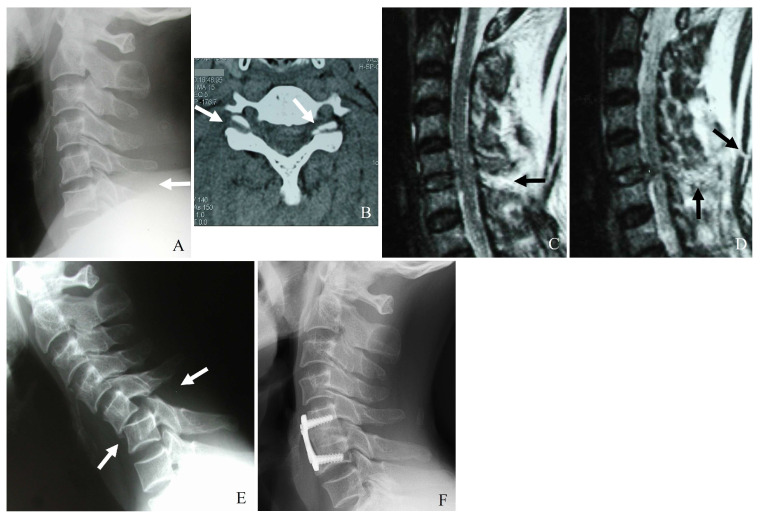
Plain X-ray (A), computed tomography (B), and magnetic resonance imaging (C,D) reveal a widening of the C5–C6 interspinous distance, a minimal fracture of the left facet, widening of the right facet joint (white arrows), traumatic herniation, and rupture of the posterior ligamentous complex at C5–C6 (dark arrows). Delayed bilateral facet dislocation of C5–C6 occurred 4 months after wearing a rigid brace (white arrows) (E). The patient underwent anterior cervical discectomy, open reduction, and fusion of C5–C6 (F).
